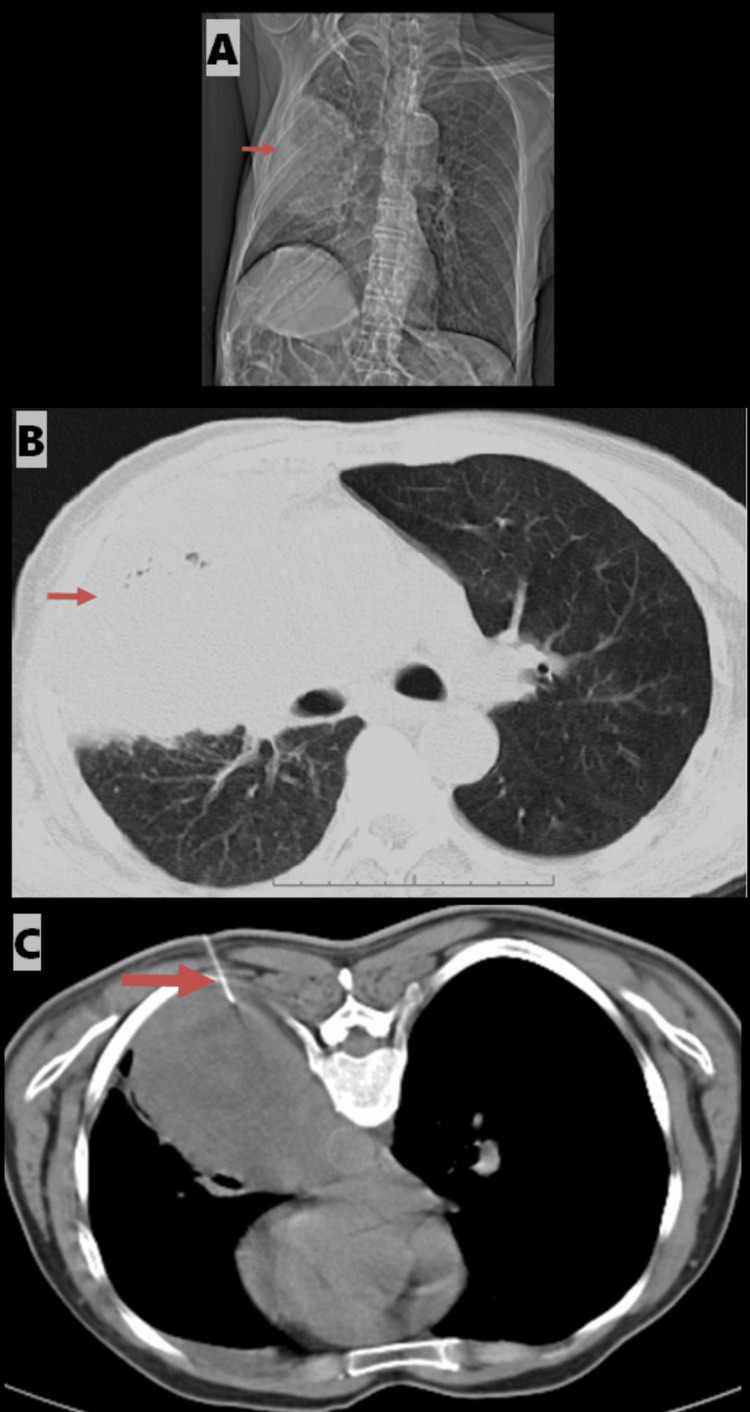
A) Topogram showing the localization of the mass in the right upper lobe (indicated by an arrow). B) Axial CT in the lung window shows a right upper lobe mass (indicated by an arrow). C) Axial CT in the mediastinal window showing the biopsy needle within the mass in the right upper lobe (indicated by an arrow).
